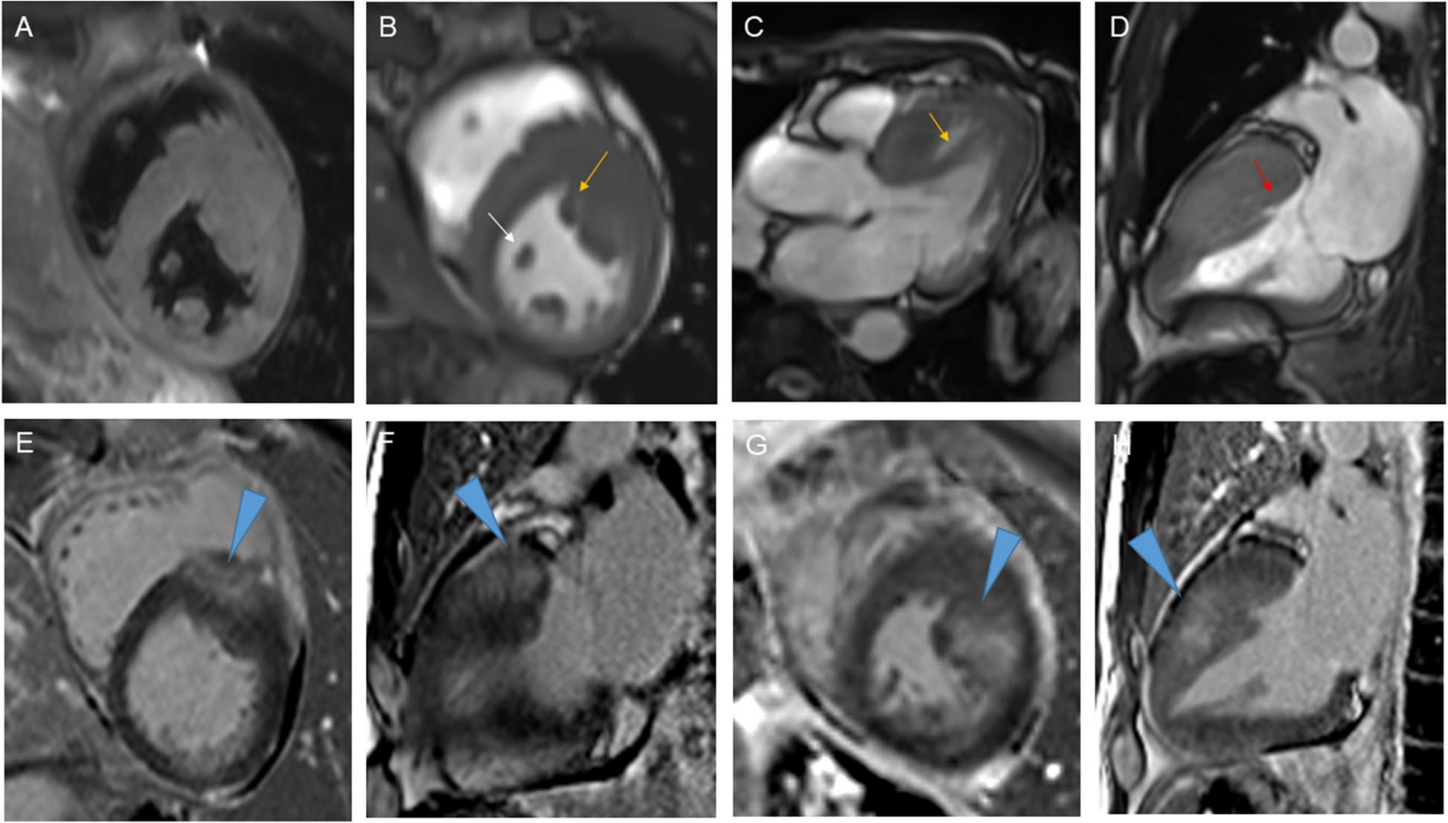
Cardiac magnetic resonance images acquired at the 10-year follow-up. T2-weighted image in short-axis orientation (A) shows the thickening of the anteroseptal wall and papillary muscle of LV. Cine images in short-axis (B), 3 chamber (C), and vertical long-axis (D) orientation demonstrate an accessory apical-basal muscle bundle inserting into the basal anteroseptum (yellow arrow), anomalous tendinous connection to the septum (red arrow), and another accessory papillary muscle (white arrow). Late gadolinium enhancement images in basal (E and F) and mid (G and H) LV show patchy enhancement in the thickened myocardium and papillary muscles (blue arrowheads). LV: left ventricle
